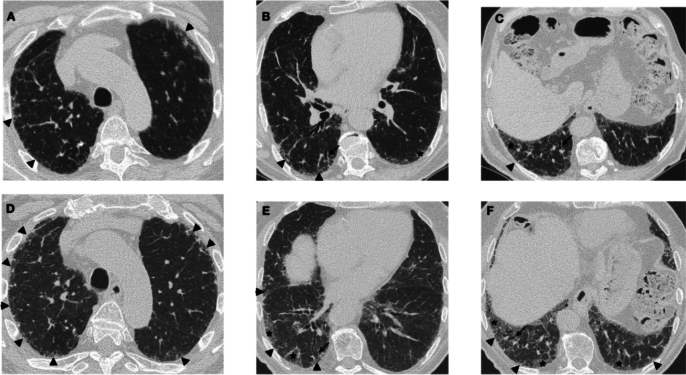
Disease trajectories in a patient with systemic sclerosis-associated interstitial lung disease and a progressive fibrotic phenotype. A–C. Images at diagnosis: Axial high-resolution computed tomography (HRCT) images show diffuse subpleural reticulation (arrowheads), subtle ground-glass opacities (*) and traction bronchiolectasis (arrows). D–F. Images at follow-up: HRCT demonstrates disease progression after 24 months due to the increased extent of ground-glass opacities (*) and reticulation (arrowheads), in association with an increased extent and severity of traction bronchiectasis (arrows)
